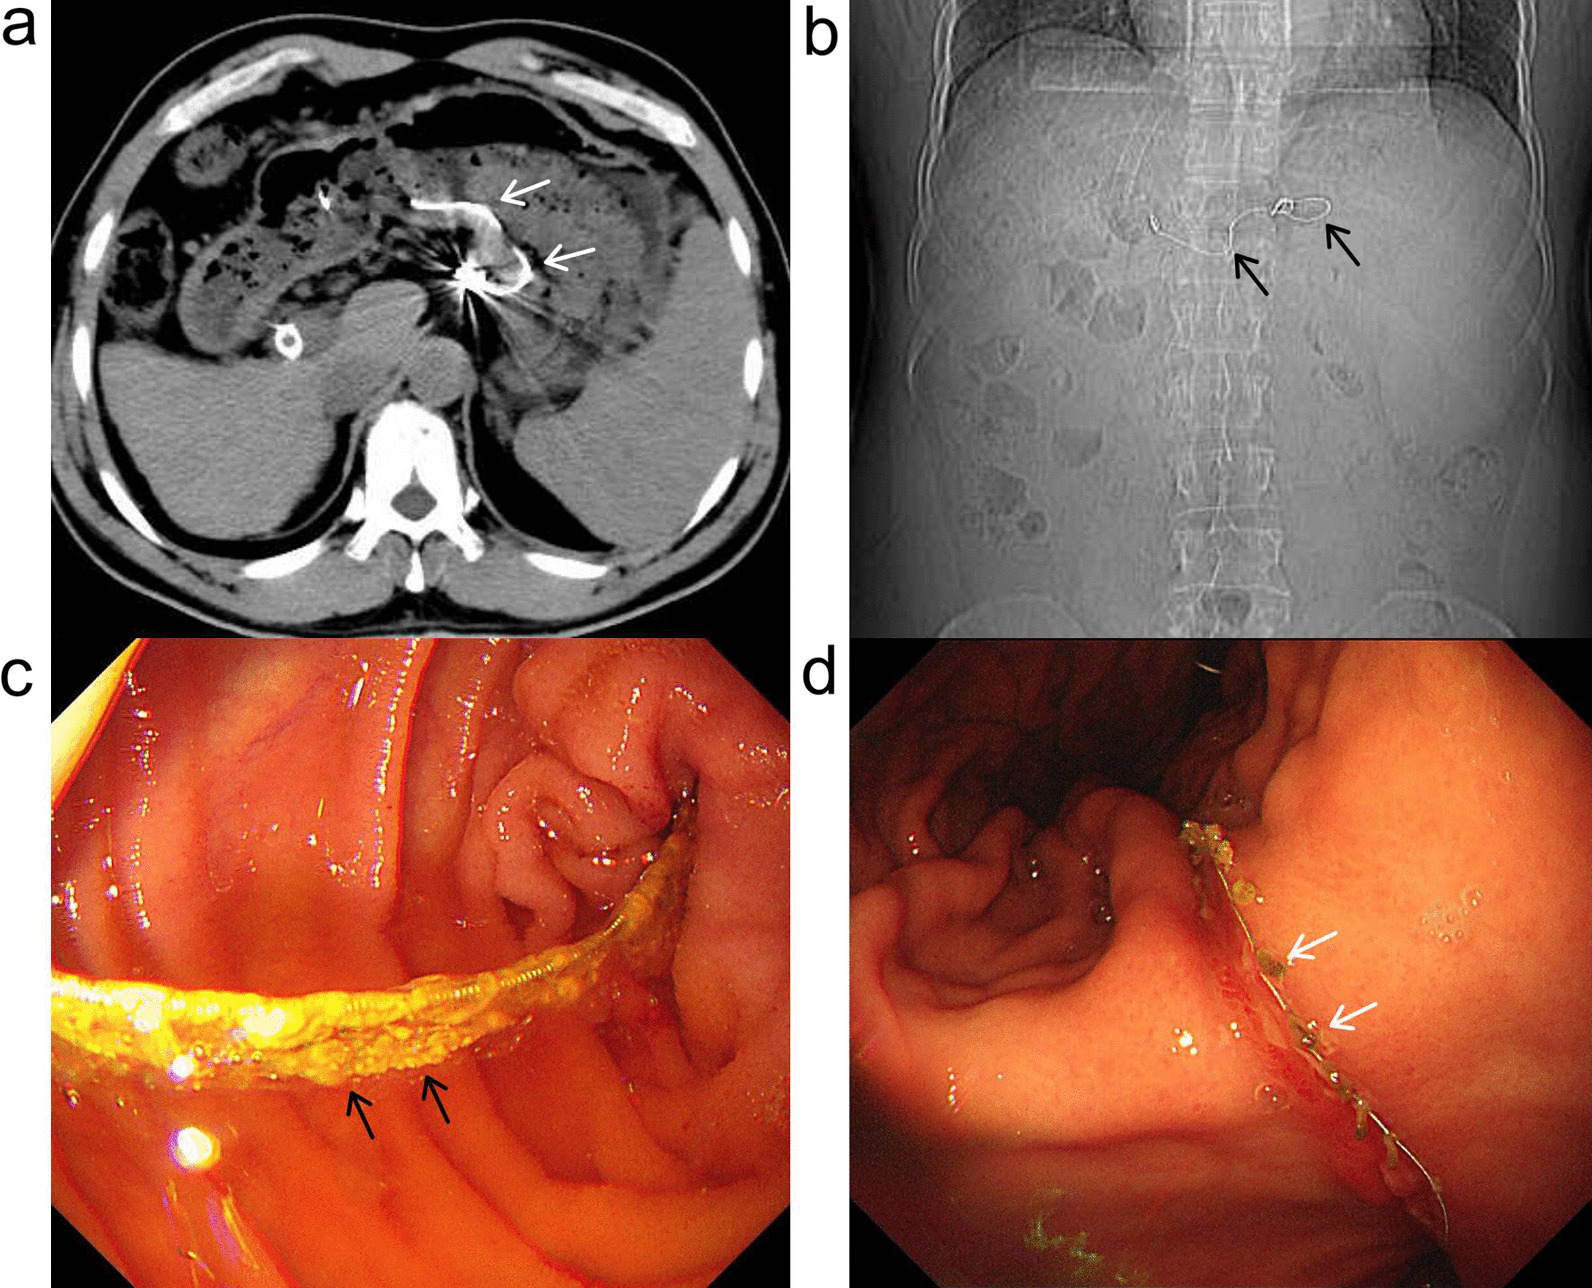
CT and EGD follow-up after TIPS. a Abdominal axial CT demonstrated the coil from the GEVs protruding into the stomach (white arrow). b Digital scout image revealing migration of the coil (black arrow). The coil was straightened, and NBCA was not visualized. c EGD view of the coil and *n*-butyl-2-cyanoacrylate migrating into the gastric wall. Adhesion of the remnants of NBCA at the surface of the coil (black arrow). d The coil extended through the gastric wall, resulting in multiple incarcerated ulcers (white arrow). CT, computed tomography; EGD, esophagogastroduodenoscopy; GEVs, gastroesophageal varices; NBCA, *n*-butyl-2-cyanoacrylate
